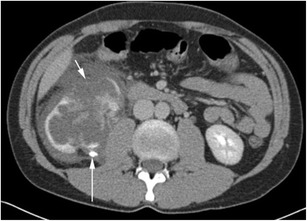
Delayed-phase CT shows fluid filling the right perinephric space in a patient following blunt trauma. The kidney has been lacerated (*short arrow*), and urinary contrast extravasation is shown posteriorly (*long arrow*)
